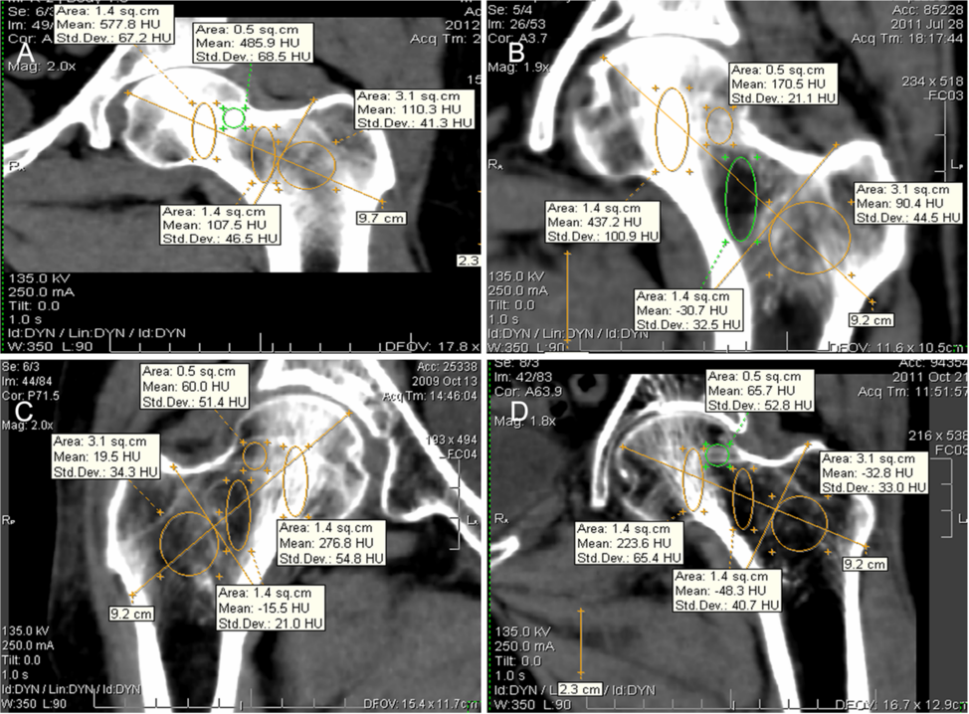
The mean CT values of four ROI for four patients with different age. (A) A 45-year-old middle age woman; (B) A 67-year-old no-fracture patient; (C) A 75-year-old woman with femoral neck fracture and (D) A 82-year-old woman with trochanteric hip fracture.
